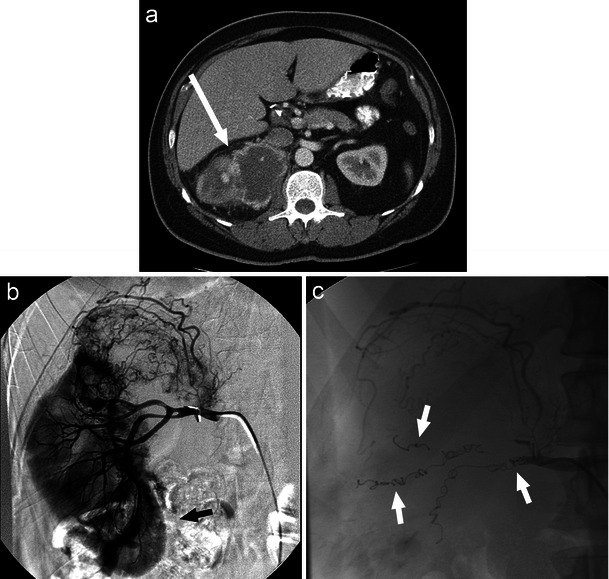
A large right RCC as demonstrated on CT (a, long white arrow) and angiographic imaging (b, black arrow). In this case, renal artery TCE (c, white arrows) allowed for tumour devascularisation prior to surgical resection
